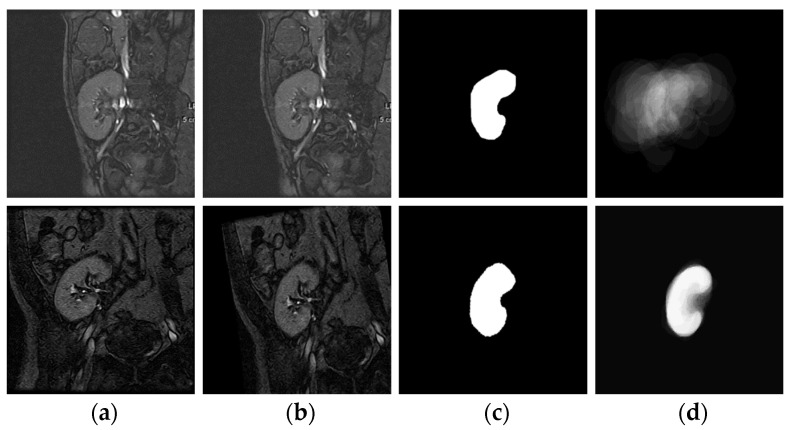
Construction of the SHP model adopting the Bayesian parameter estimation method. Columns (a,b) show non-registered and registered DCE-MRI kidney images. Column (c) shows ground-truth segmentations. Column (d) depicts the SHP-model built before (top) and after (bottom) affine registration.
